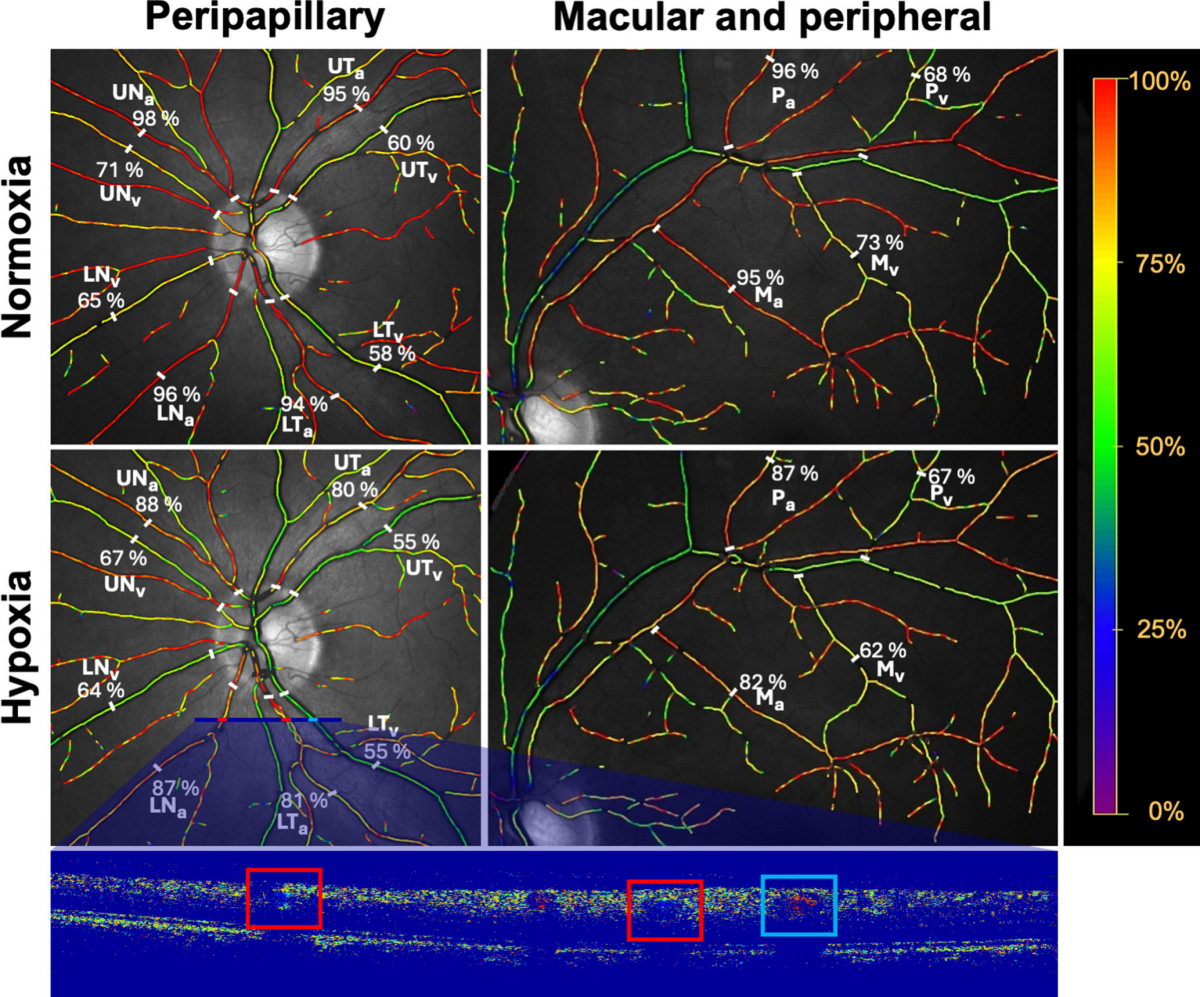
Oximetry images centered on the optic disc (*left*) showing the four selected peripapillary arterioles and venules and the upper temporal vessels (*right*) during normoxia and systemic hypoxia. The colors of the vessels represent the oxygen saturation as shown on the right vertical bar. The selected segments are delimited by *white bars* with the oxygen saturations shown at the end of each segment. UT, upper temporal; UN, upper nasal; LT, lower temporal; LN, lower nasal; P, peripheral; M, macular. Subscripts a and v denote arterioles and venules, respectively. The lower bar shows the Doppler OCT scan covering the lower nasal and temporal arterioles (*red rectangle*) and the lower temporal venule (*blue rectangle*).
